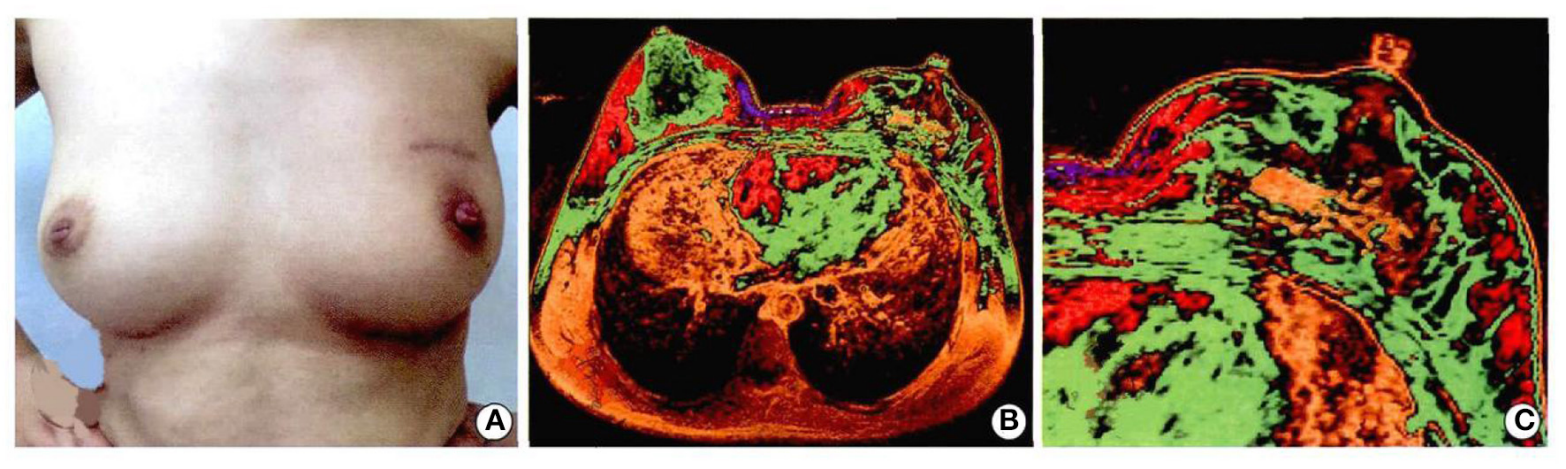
Patients with breast cancer underwent left breast segment resection + 3D printing implant + axillary lymph nodes dissection. The breast appearance and follow-up of 9 months were as follows: (A) figure: cosmetic effect of breast; (B) magnetic resonance imaging of breast; (C) magnetic resonance imaging of implant. Written informed consent was obtained from the patient for the publication of any potentially identifiable images or data included in this article (26).
